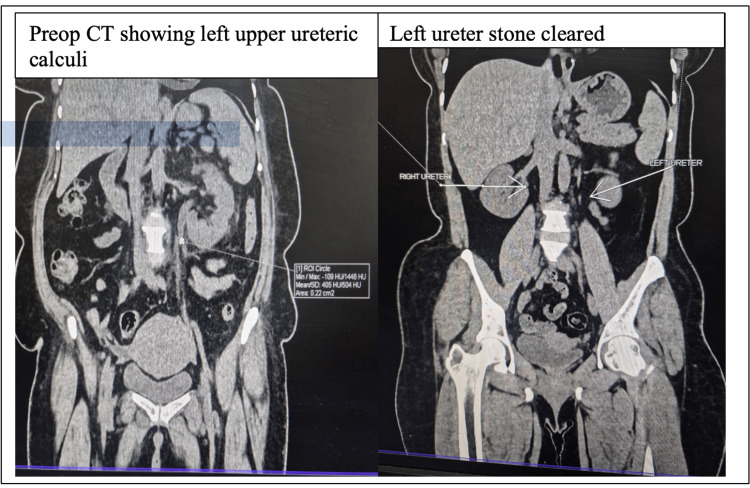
Low-density ureteric calculus. Preoperative coronal non-contrast computed tomography (NCCT) image demonstrating a low-density ureteric calculus with a circular region of interest (ROI) measurement (mean Hounsfield unit (HU) value, 405; area, 0.22 cm²). This patient achieved complete stone clearance at one month.
